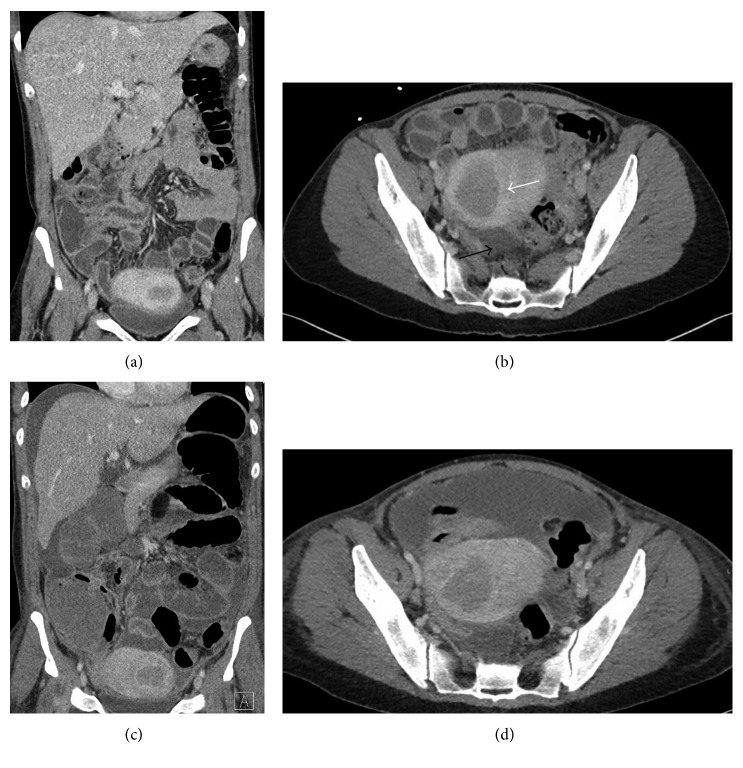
Initial CT scan upon presentation (a, b) with uterine fibroids (white arrow) and minimal free fluid (black arrow). CT scan four days after admission with worsening ascites (c, d).
